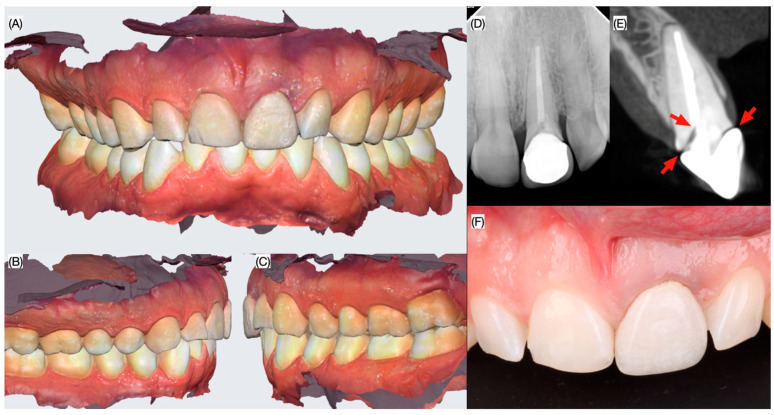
Initial situation. Intra-oral scan (A) frontal, (B) right and (C) left side view, (D) peri-apical radiograph, (E) interproximal view with CBCT scan showing the fractured lines and (F) frontal view.
